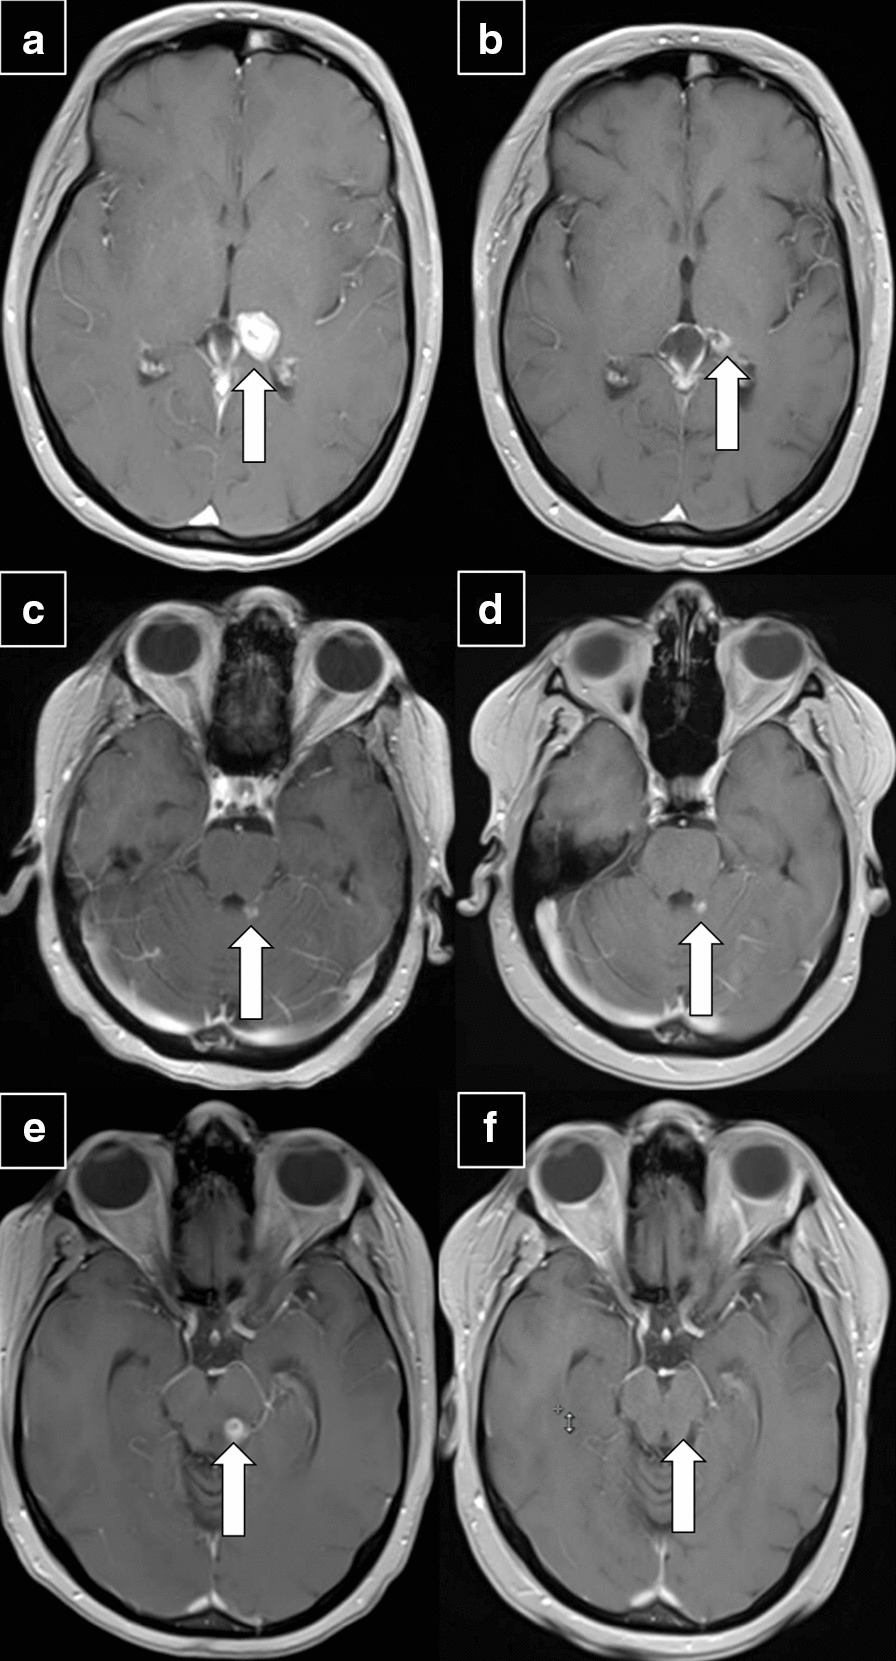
T1-weighted, post-contrast MRI brain images of a: a left thalamic lesion in July 2014 (arrow) treated with fractionated stereotactic radiotherapy (3000 cGy in 5 fractions) and b post-treatment images in August 2015; c small contrast-enhancing lesion in the left cerebellar hemisphere (arrow) in December 2014 which was treated with stereotactic radiation to the left cerebellar lesion in January 2015 and d post-treatment images in August 2015; e a 1.3 × 1.0 × 1.9 cm lesion involving the posterior inferior left thalamus (arrow) extending inferiorly to involve the left midbrain tectum in March 2015 treated with whole brain radiation therapy with 30 Gy over 10 fractions completed on April 2015 and f post-treatment images in August 2015.
